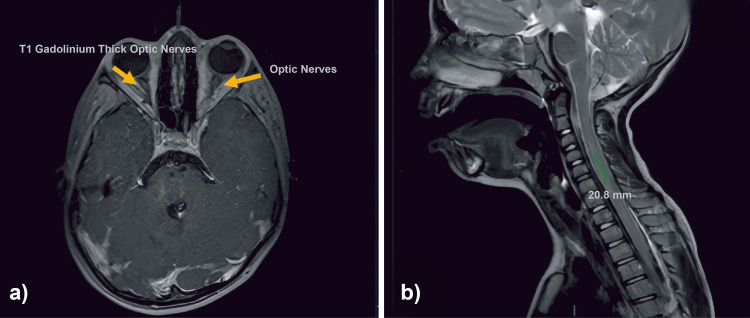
Representative images from the patient's magnetic resonance imaging (MRI). a) Axial and coronal MRI of the orbits. On the axial T1‑weighted post‑contrast sequence, bilateral intraorbital optic nerve enlargement with smooth, longitudinal enhancement is observed, confined to the intraorbital segments on this slice, with no visible involvement of the canalicular, intracranial, or chiasmal portions. On the coronal T2‑weighted sequence, diffuse thickening of both optic nerves (>5 mm) is evident (arrows). In the contrast‑enhanced phase, bilateral optic nerve enhancement is present, and a target sign is noted. Additional supratentorial lesions are visible, with probable thalamic involvement, supporting multifocal CNS demyelination. b) Sagittal cervical spine MRI. The T2‑weighted sequence demonstrates a 20.8 mm centrally located hyperintense lesion, corresponding to approximately 1.5 vertebral segments, involving the anterior and central portions of the spinal cord. Additional longitudinal hyperintensities are visible from C3 to C5, spanning more than two vertebral bodies. These lesions enhance following contrast administration and represent areas of myelin involvement. No associated cord expansion is observed.
